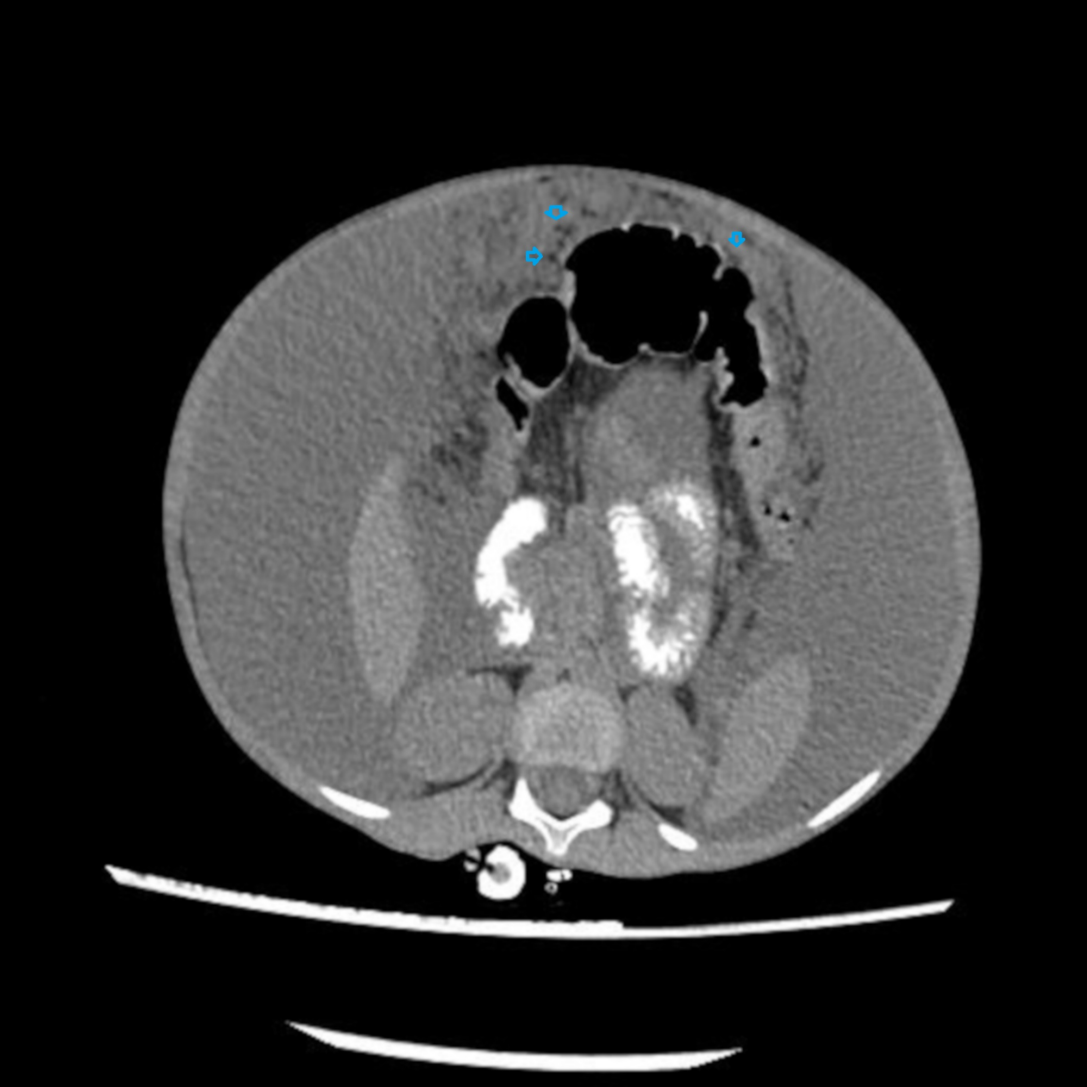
Computed tomography (CT) scan of abdomen with contrast showed omental thickening and nodularity (arrows).
